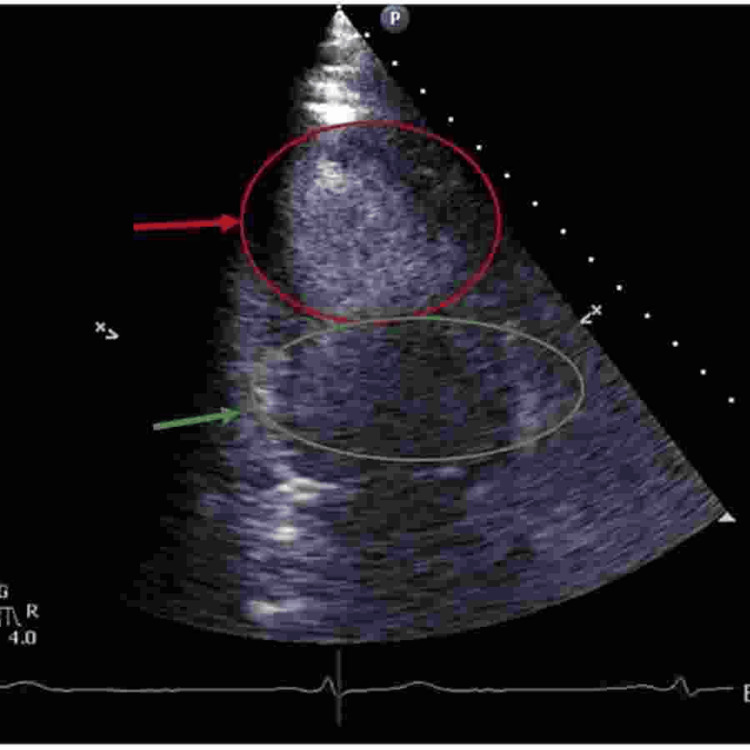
This is the same contrast TTE apical view of the left ventricle during systole; the red arrow indicates apical contraction of LV during systole, and the green arrow shows hypokinesis of the base of LV. LV: Left Ventricle, TTE: Transthoracic Echocardiogram
